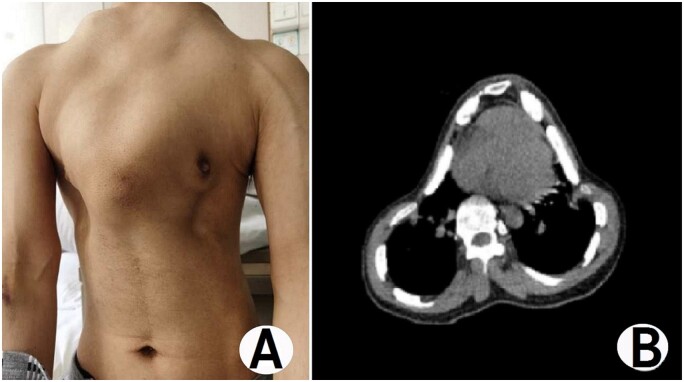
The appearance of the chest wall and computed tomography images of the patient. Preoperative chest wall appearance (A) and preoperative chest CT image (B).
